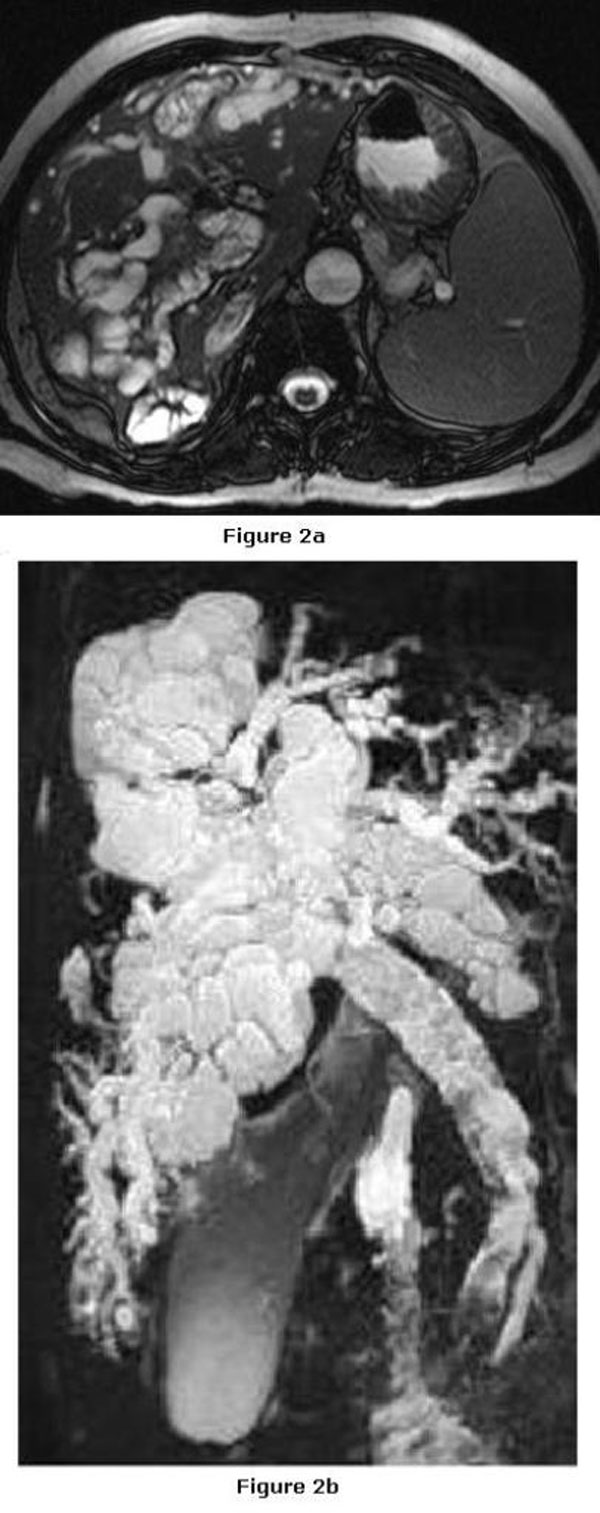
Axial T2-weighted MRI (a) and MRCP (b) demonstrating extensively dilated hepatic bile ducts and common bile duct filled with hydatic cyst material seen as hypointense lesions.
